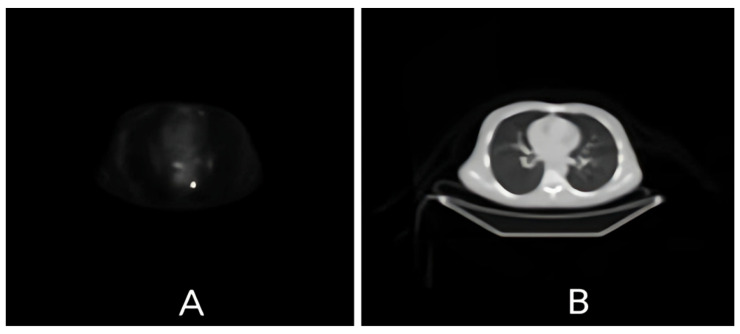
Real-time PET (A) and CT (B) images acquired from the same patient dataset. PET provides functional metabolic information, whereas CT offers high-resolution anatomical detail. These paired images were used as inputs for subsequent registration experiments.
